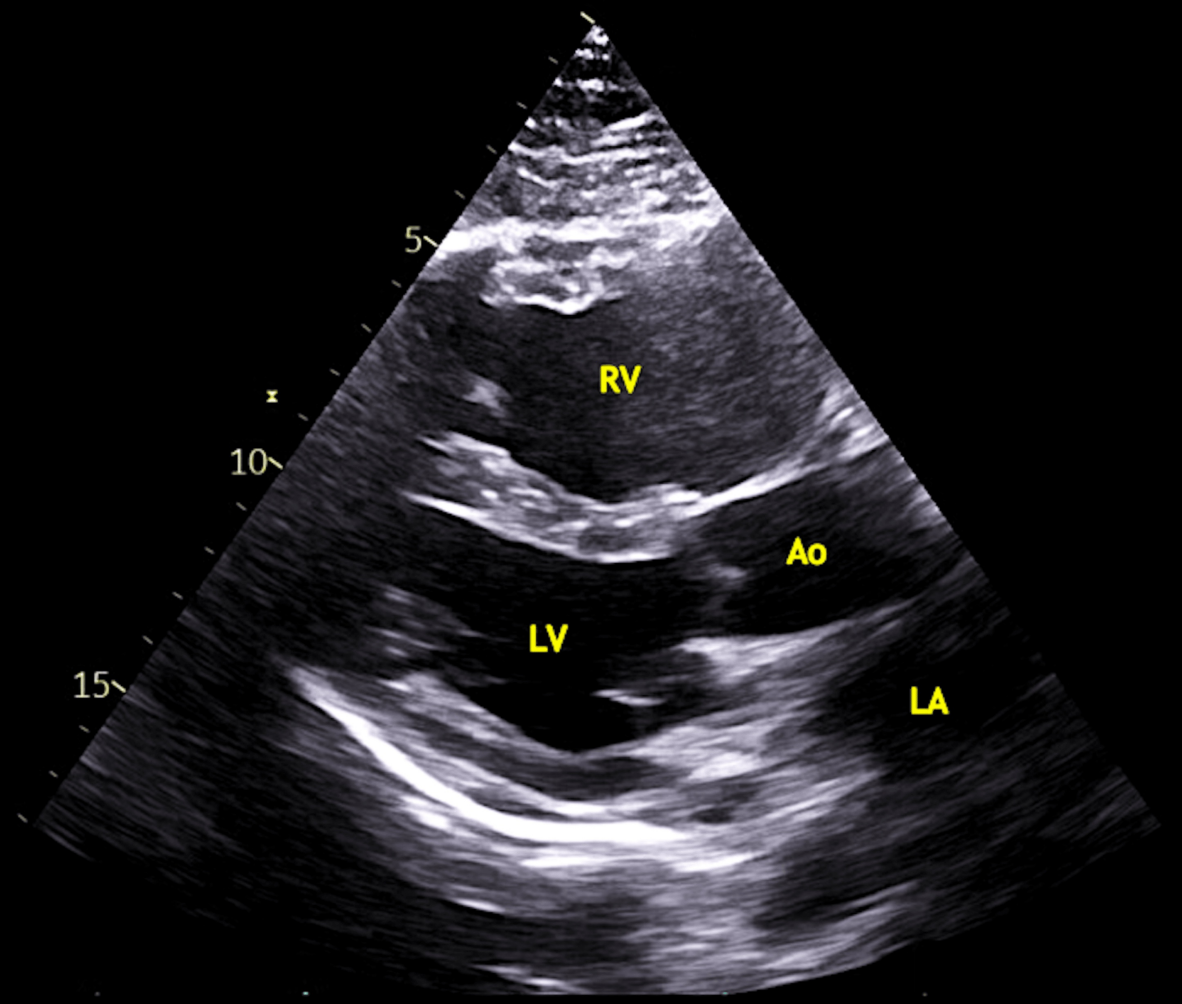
The parasternal long axis view again demonstrates right ventricle dilation with an RV/LV ratio >1 RV - right ventricle; LV - left ventricle; Ao - aorta; LA - left atrium Original image by authors.
